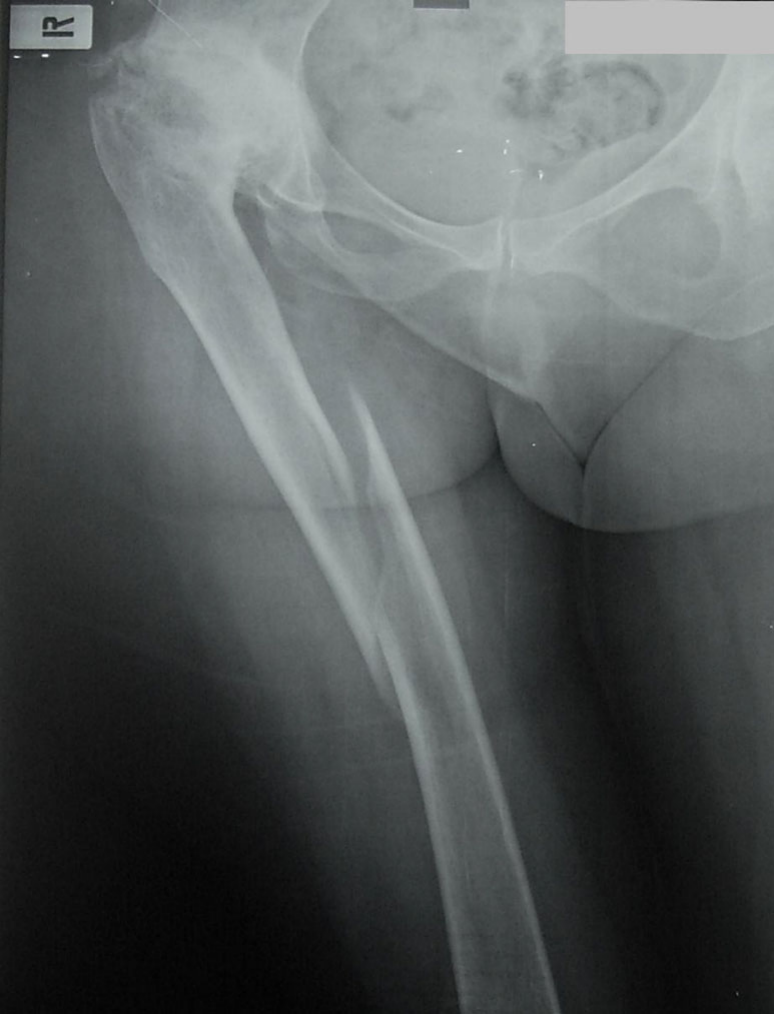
Initial post traumatic anteroposterior X-ray of the femur. Hip dysplasia with severe arthritis is recognized. An oblique mid shaft fracture is revealed
